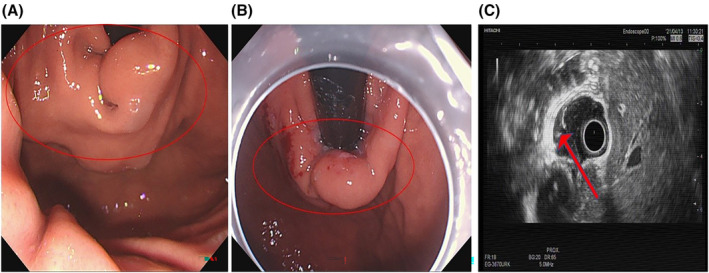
Endoscopy. (A) An endoscopic view from 2016: Protruding mass with a smooth surface was observed in the esophagogastric junction. (B) An endoscopic view from 2021: Protruding mass with slightly hyperemic surface was observed in the esophagogastric junction. (C) Endoscopic ultrasonography from 2021: A mass was derived from the musculus propria and surrounded about half of the cavity, with irregular shape.
